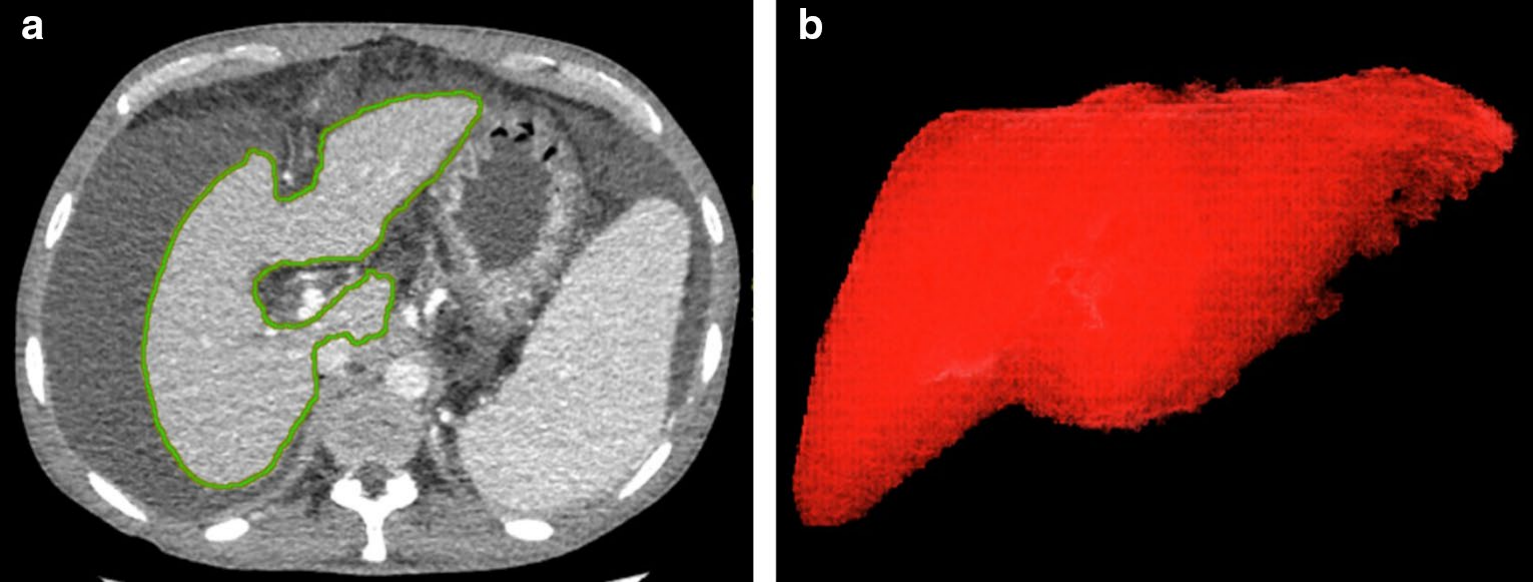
Liver volume was measured on images of enhanced CT at venous phase (2a), with software (AW 4.7, total liver and segment separation), and finally the whole liver volume would be calculated automatically and shown with volume rendering (2b).
